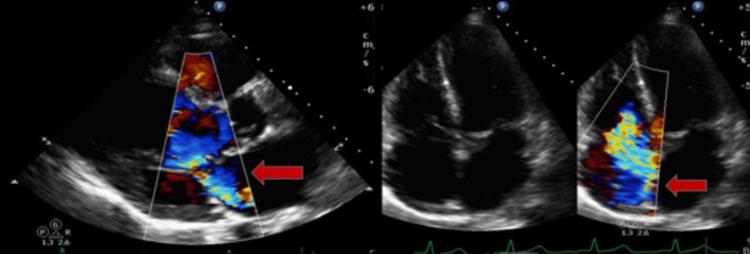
TTE with parasternal long axis view (left) showing moderate mitral valve regurgitation jet (red arrow); apical 4 chamber view (right) showing moderate to severe TR
